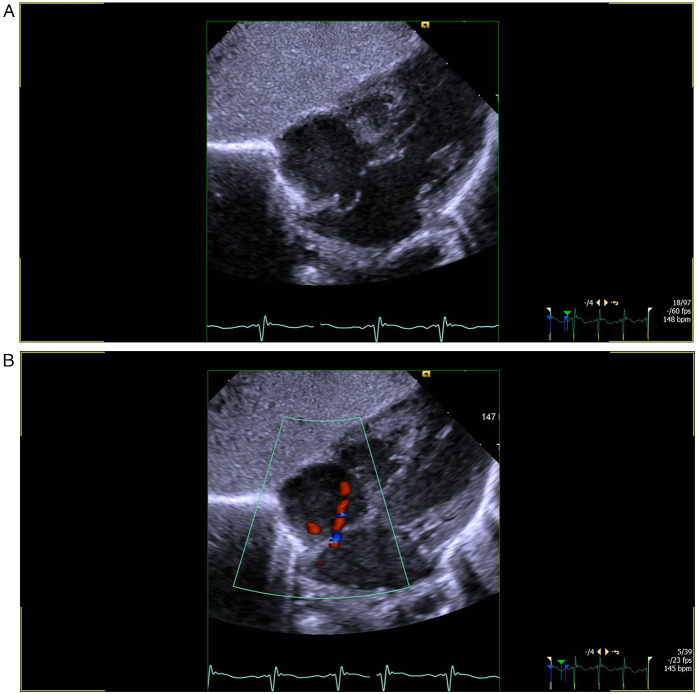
(A, B) Transthoracic echocardiography, subcostal four-chamber view, of a thin interatrial septum with a restrictive FO.
